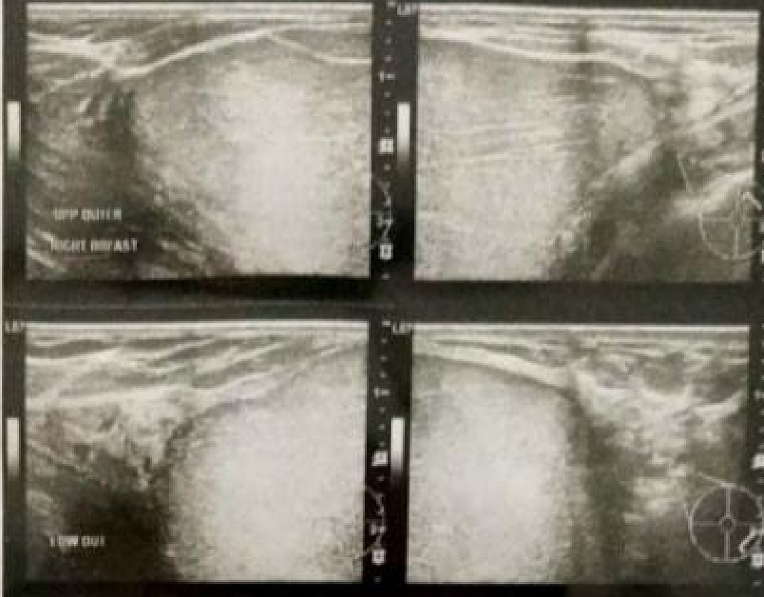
Ultrasound examination showed homogeneous solid lesion, oval in shape with firm boundaries, encapsulated in the right breast quadrant.
